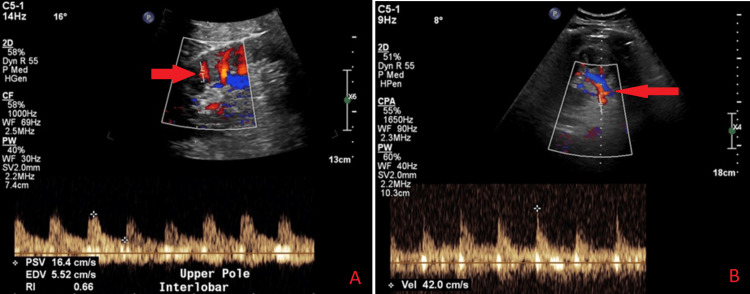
Post-stent Doppler ultrasound examination of the left kidney shows preserved perfusion (panel A), as well as the patent distal left main renal artery with a normal peak systolic velocity (panel B). The interlobar arteries showed a normal peak PSV, EDV and RI of 16.4 cm/s, 5.52 cm/s, and 0.66 in the upper pole; 19.4 cm/s, 6.6 cm/s, and 0.66 in the middle pole; and 24.6 cm/s, 8.46 cm/s, and 0.66 in the lower pole, respectively. The visualised distal segment of the main left renal artery was patent with a normal peak systolic velocity of 43.1 cm/s.
